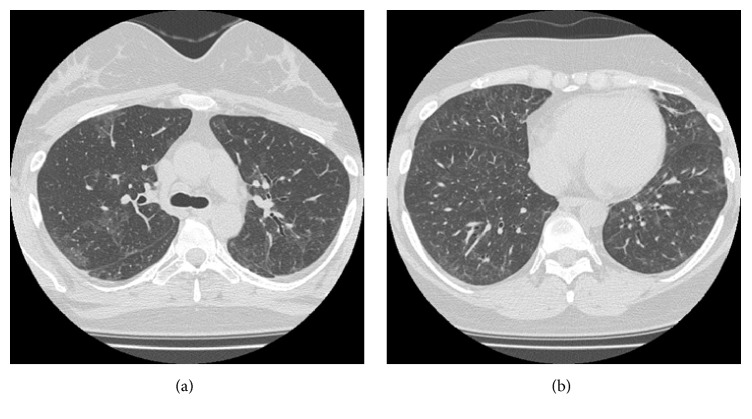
Chest CT scans showing the patient's middle (a) and lower (b) lung fields after two months from the initiation of treatment. The pulmonary infiltrates had disappeared.
